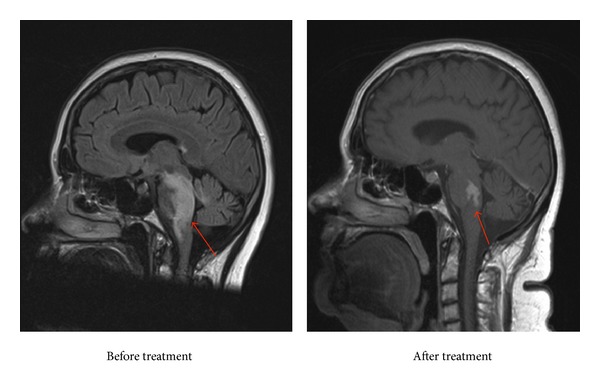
MRI brain demonstrating brainstem and cerebellar lesions before and after 2 cycles of with high-dose methotrexate.
